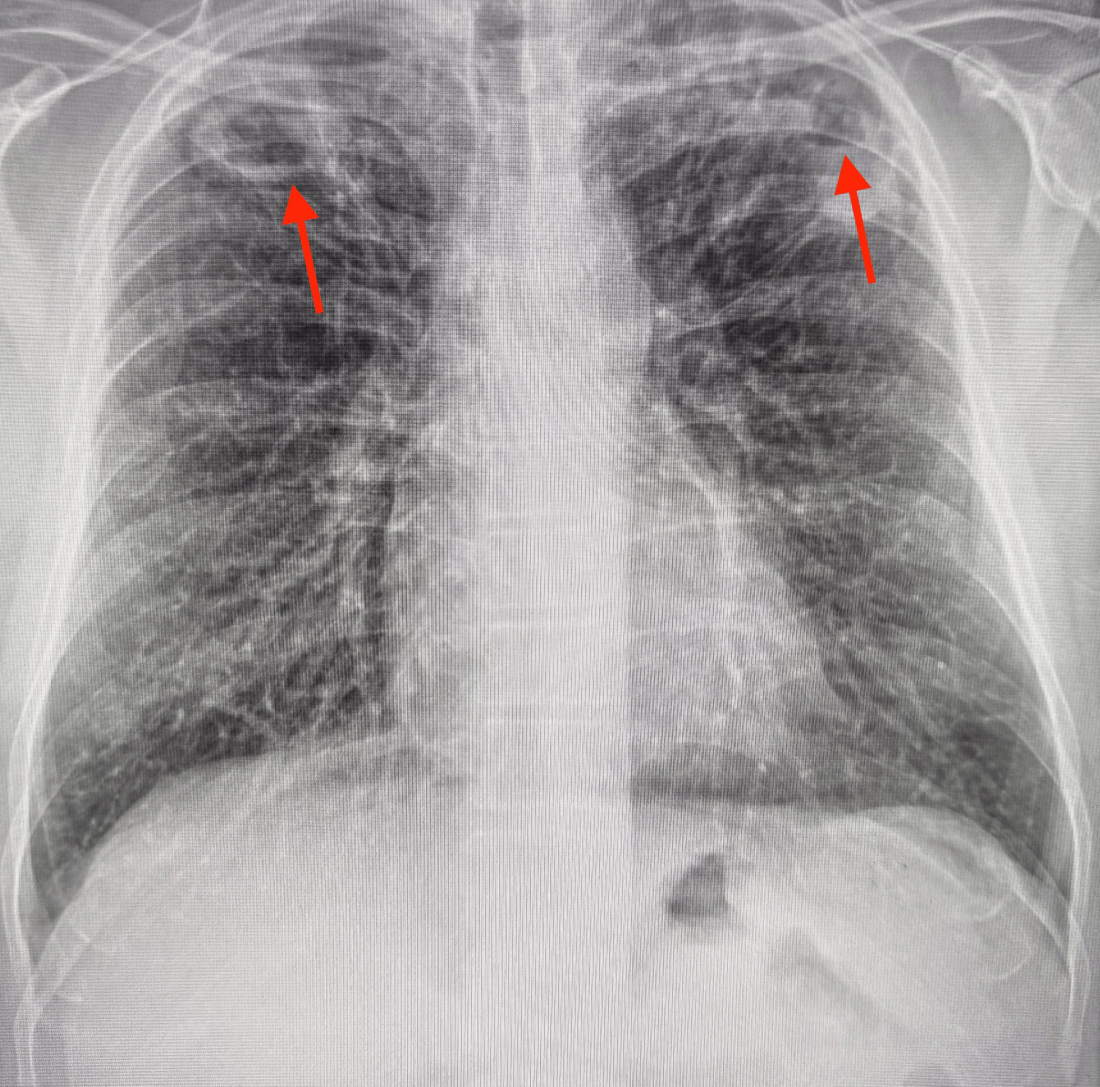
Anterior-posterior chest X-ray showing bi-apical opacities with cystic lucencies (arrows)
